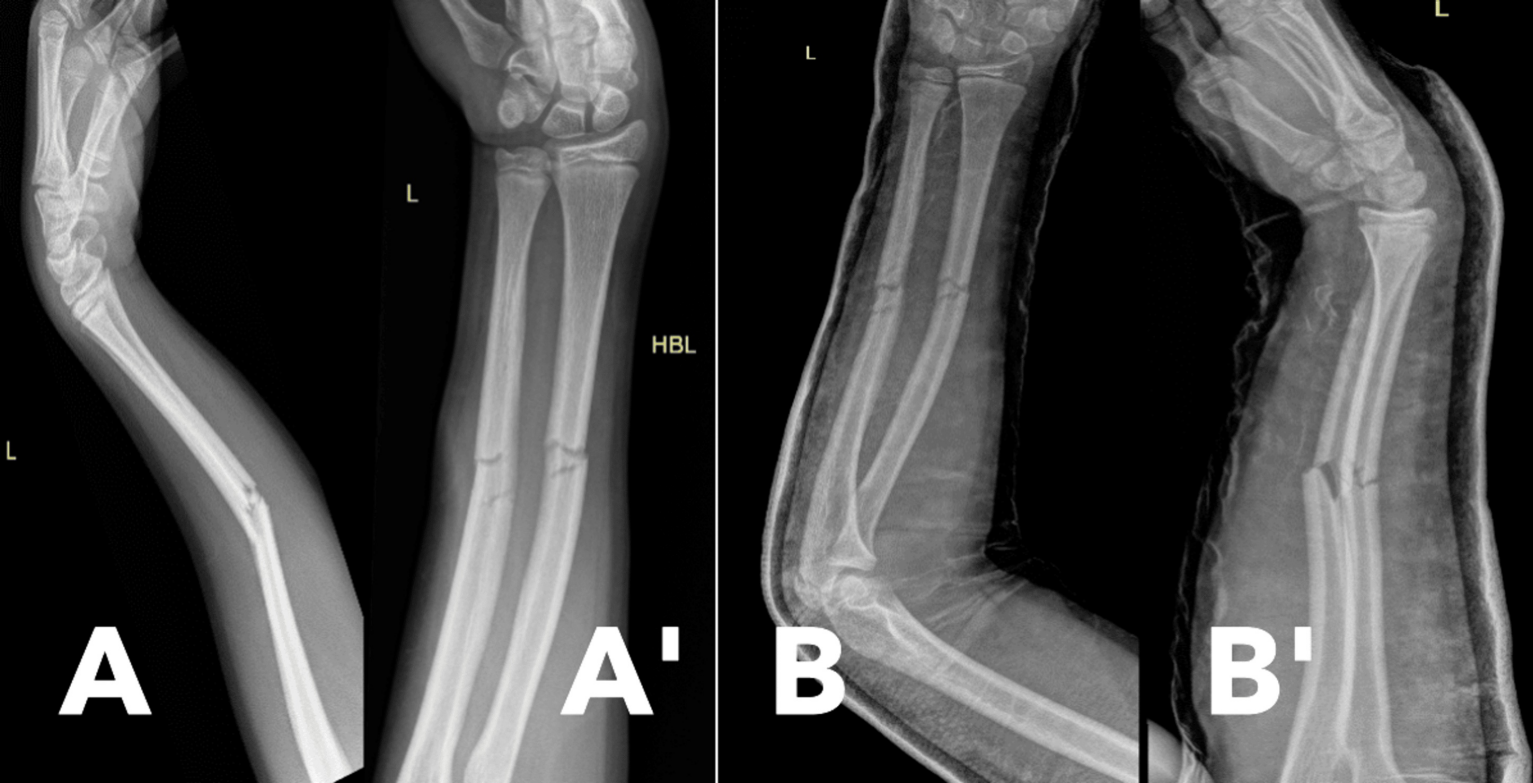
Initial radiographs (A) Lateral view of initial fracture; (A') AP view of initial fracture; (B) AP view of initial fracture after application of a back slab; (B') Lateral view of initial fracture after application of a back slab
